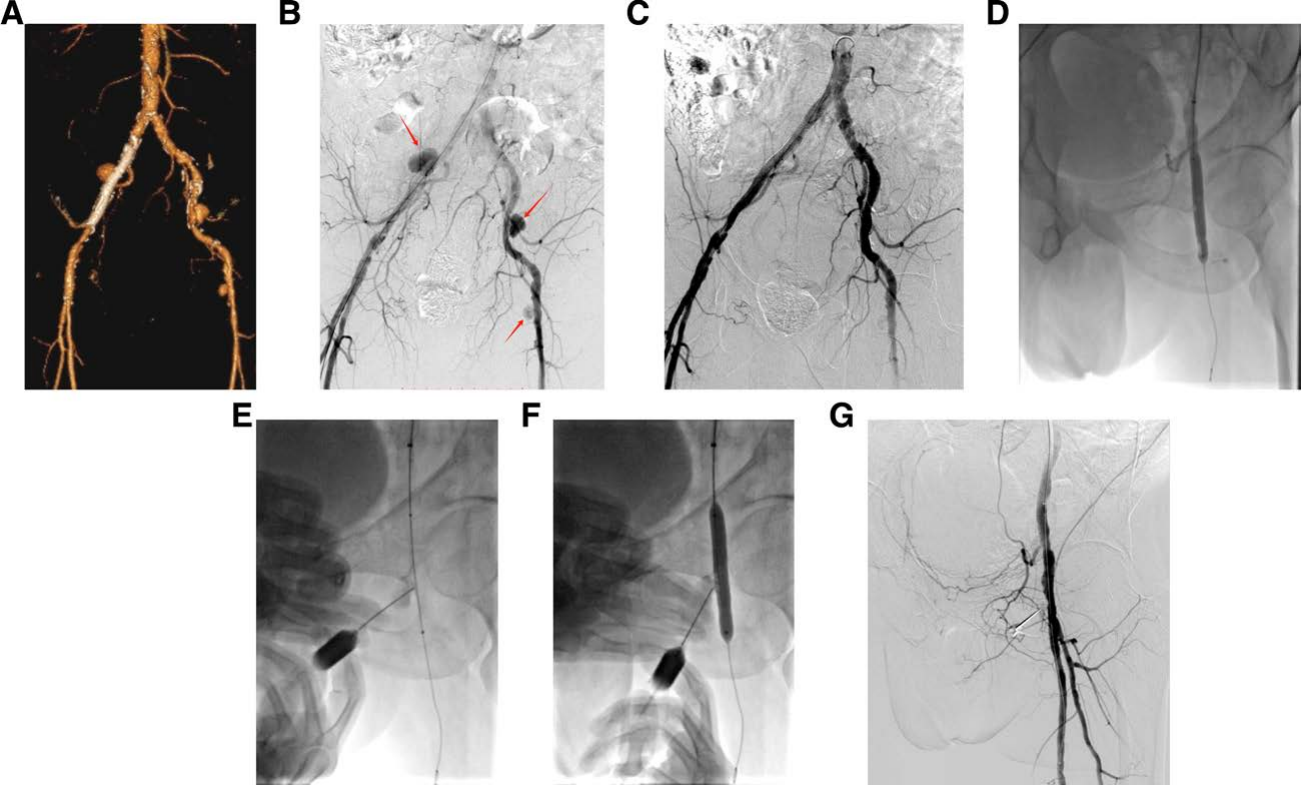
(A) Preoperative CTA showed that the patient’s bilateral external iliac arteries and left common femoral artery pseudoaneurysms were formed, and atherosclerosis was obvious; (B) We performed imaging in the abdominal aorta, confirming the location and morphology of the 3 pseudoaneurysms; (C) We first dealt with bilateral external iliac artery pseudoaneurysms, and bilaterally implanted a 9 mm × 5 cm overlying stent (Viabhan, GORE) anchored to the aneurysmal rupture. The contrast review suggested that bilateral external iliac artery aneurysms were not visualized; (D) after using a balloon to seal the neck of the left FAP aneurysm, the FAP was not visualized by overhead manual push contrast; (E) the balloon was withdrawn, the left FAP was punctured, and contrast was injected to confirm that the tip of the needle was in the FAP; (F) the balloon was filled and sealed the neck of the FAP aneurysm, and thrombin + contrast were injected to make the aneurysm cavity visualized, and the sealing was maintained for 3 minutes; (G) contrast review did not show the left FAP visualization. CTA = computed tomography angiography, FAP = femoral artery pseudoaneurysm.
